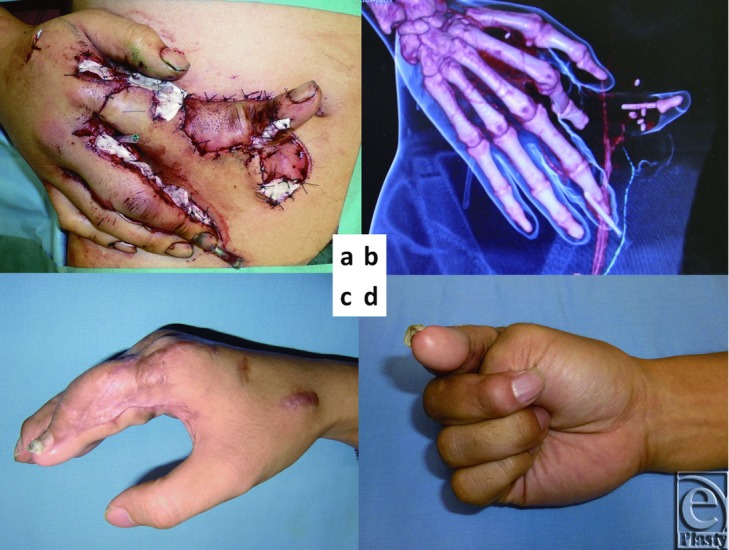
Amputation resulting from printing machine accident in a 29-year-old man. (a) The digital artery of the index finger was anastomosed to the deep inferior epigastric artery perforator (DIEP) at the level of the distal interphalangeal joint. (b) Computed tomographic angiography demonstrating connection between the DIEP and the digital artery. (c) Six months after ectopic implantation. (d) Grip position.
